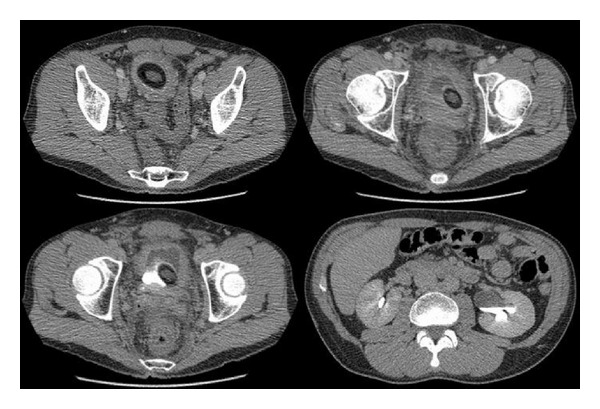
CT scan of the pelvis, demonstrating the toilet brush and surrounding fluid. There is a left-sided hydronephrosis (right, lower picture).
